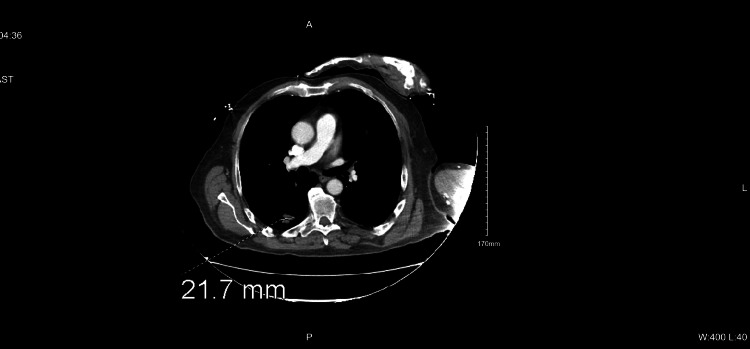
CT of the lung showing the second of two apical right lower lobe tumors CT: computed tomography
